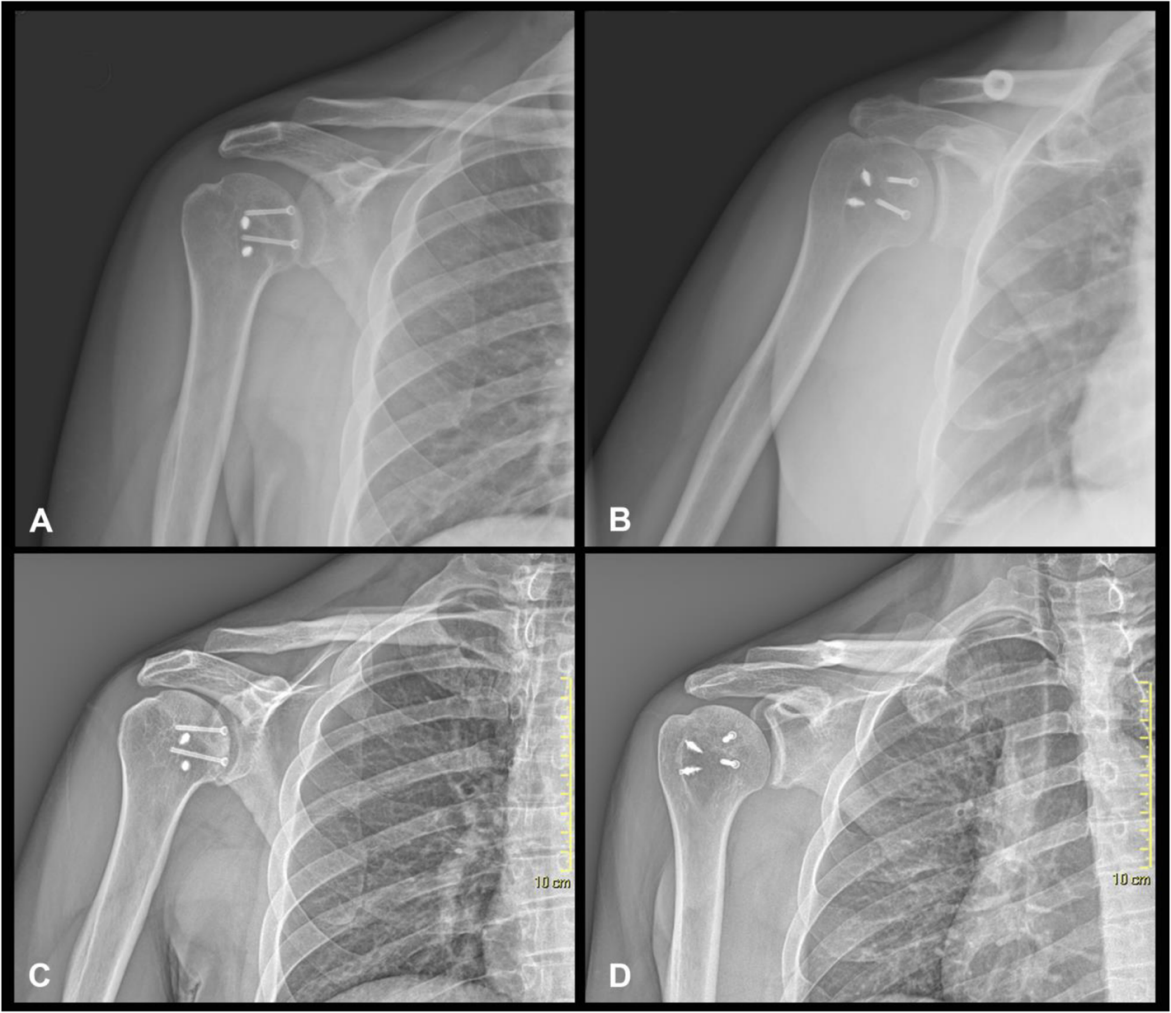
Standard shoulder Anteroposterior X-Ray of the same patients taken post-operative (A,B) and at 65 months follow-up (C,D). Osteoarthritis (OA) was graded according to Samilson-Prieto Score (Grade I in this case)
